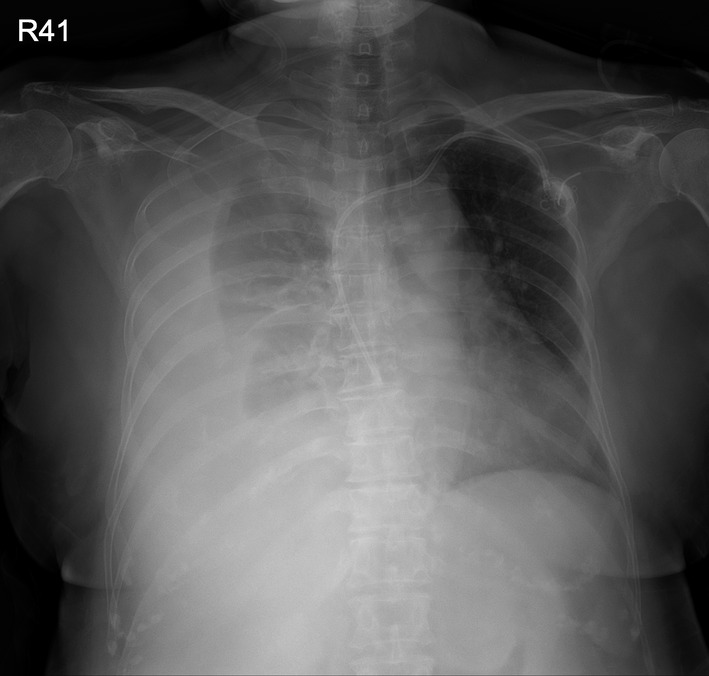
Chest roentgenogram showed right‐sided massive pleural effusion after thoracentesis
